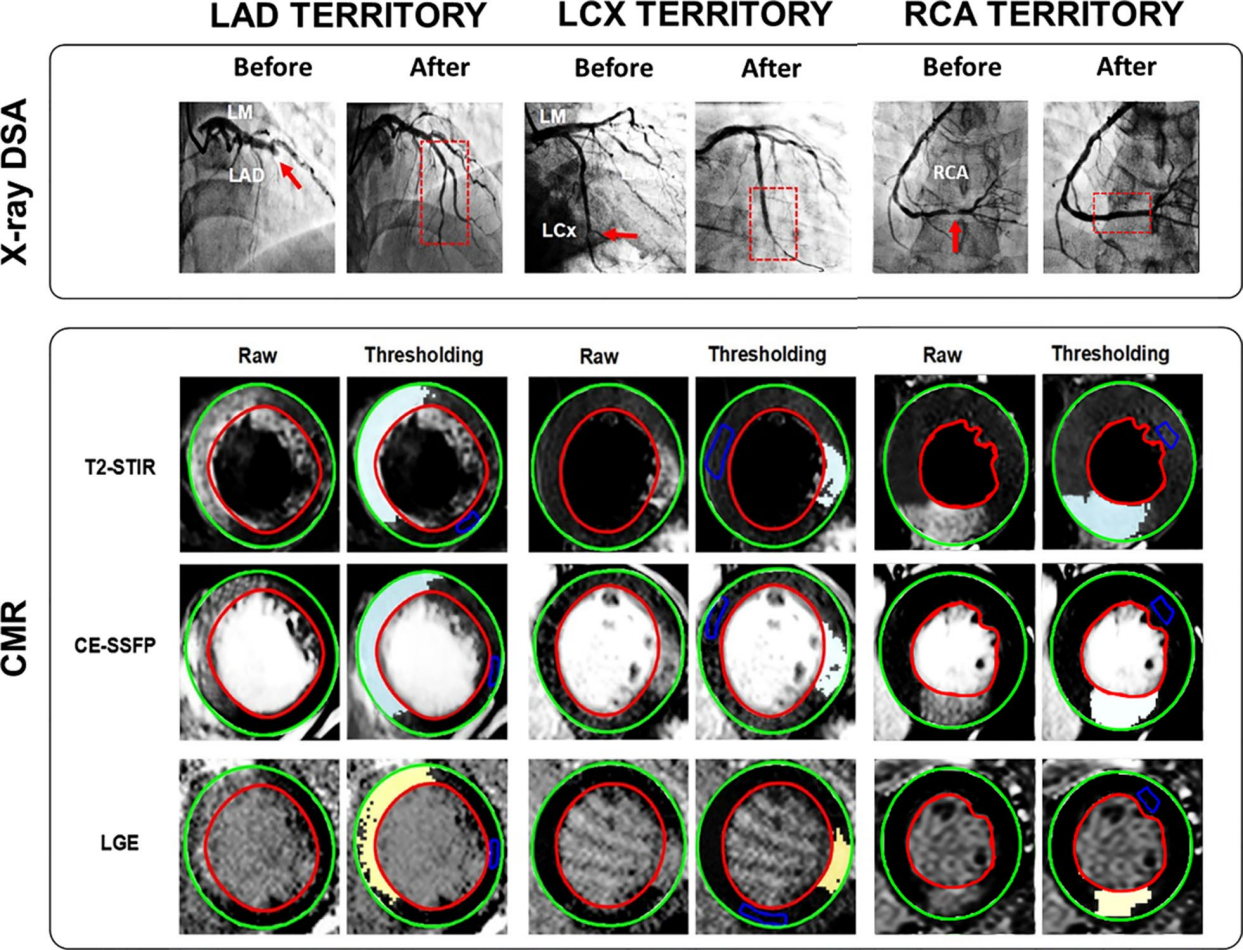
Representative Images from STEMI Patients with LAD, LCx and RCA Myocardial Infarctions. X-ray Digital subtraction angiography (X-ray DSA) images acquired before and after PCI, along with corresponding short-axis myocardial T2-STIR and CE-SSFP showing at-risk regions and infarct territories (LGE) acquired at 3T are shown. On X-ray DSA images, the red arrows refer to occlusive or substantially narrowed vessels, and the red box with dotted line demonstrates the re-establishment of blood flow following recanalization of the corresponding vessel. On CMR images, epicardial and endocardial borders are delineated by green and red borders, respectively. The left columns shows the raw images and the right columns shows the same images processed using semi-automatic threshold-based signal detection. The edematous myocardium delineated by the T2-STIR and CE-SSFP images are shown as a region enclosed by the pale blue areas and irreversibly damaged myocardium is identified on LGE images as yellow areas
